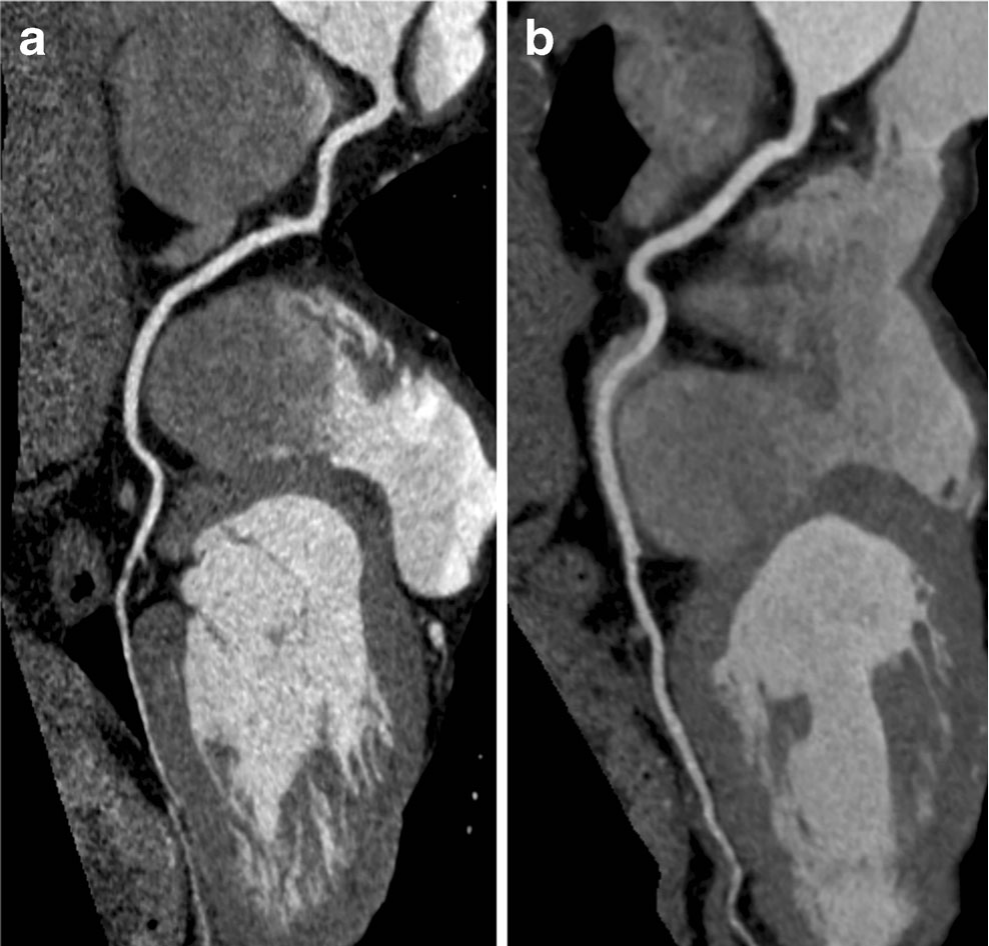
The right coronary artery in patients of similar BMI scanned with Triple-Rule-Out CT angiography at 100 kV (a) and 120 kV (b)
